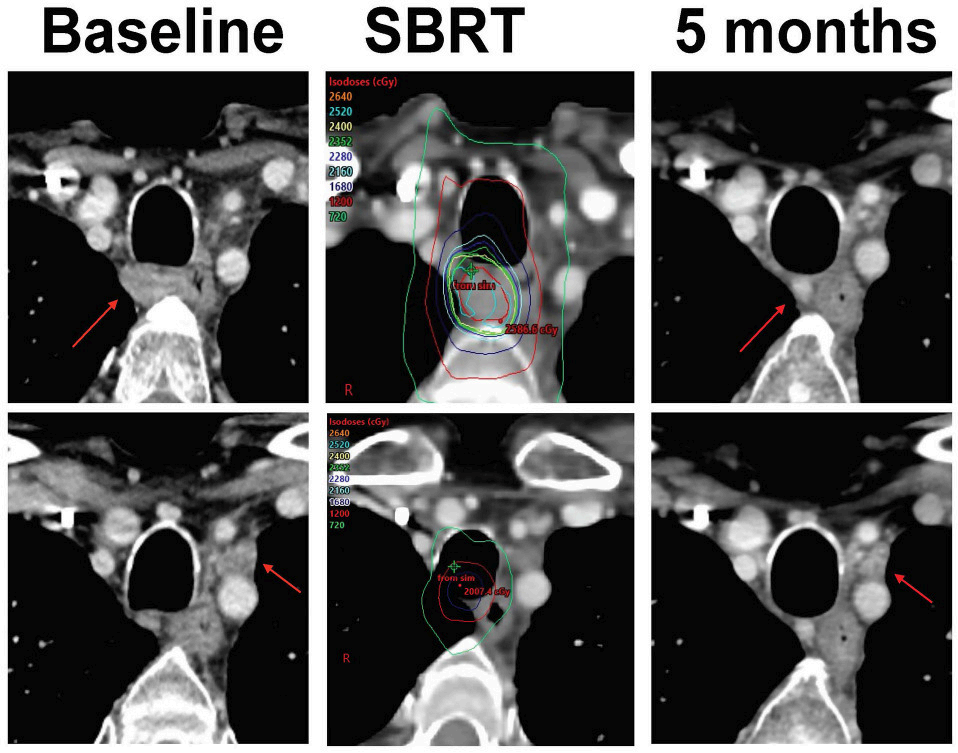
CT imaging of patient with Stereotactic Body Radiation therapy (SBRT) plus ipilimumab and nivolumab. Resolution of right sided tracheoesophageal lymph node and enlarged node between the left common carotid and subclavian arteries. The patient had no new lesions and no measurable disease by RECIST criterion consistent with a complete response. Patient has remained in complete response with continued decrease in size of these lymph nodes as of most recent imaging 2 years after his initial treatment with palliative RT combined with nivolumab.
